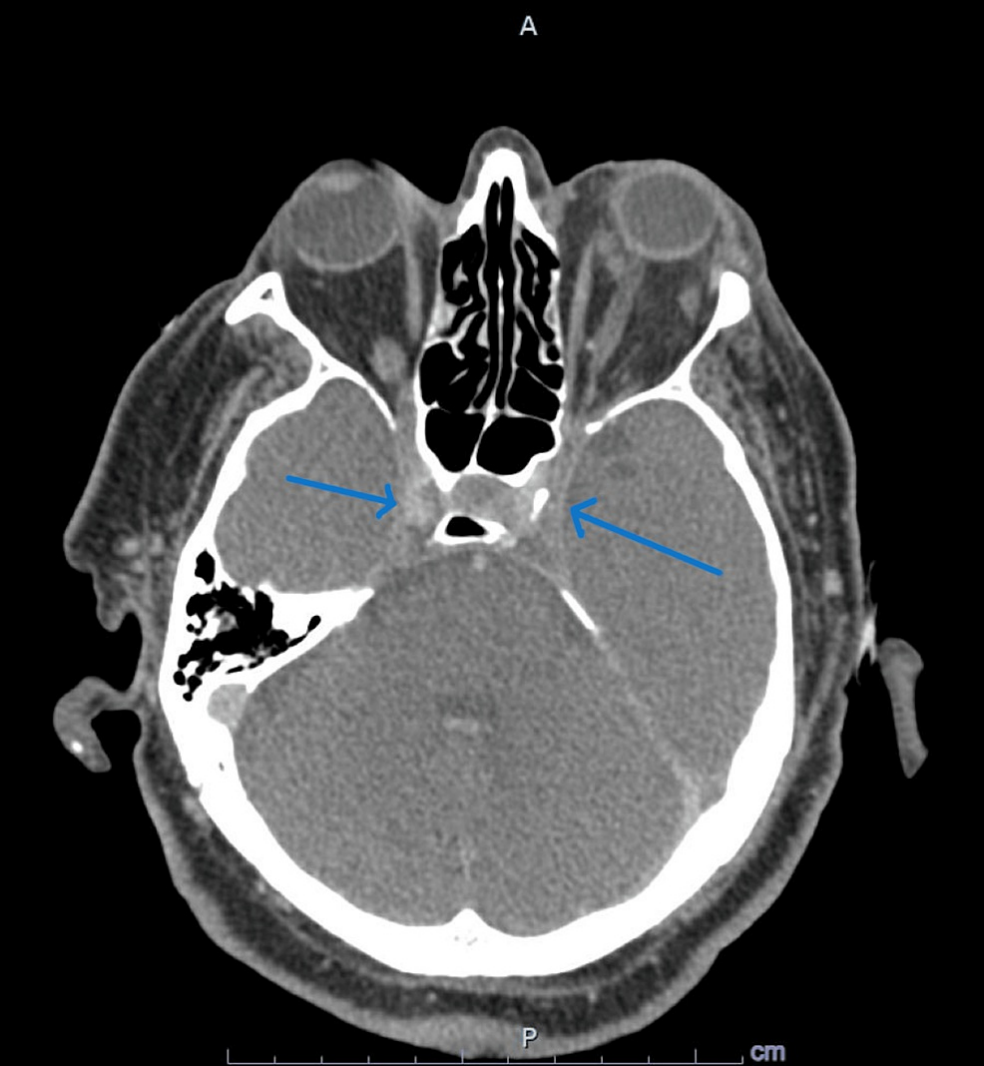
CT venogram of the head showing dilated ophthalmic veins with diminished enhancement in both cavernous sinuses compatible with bilateral cavernous sinus thrombosis
